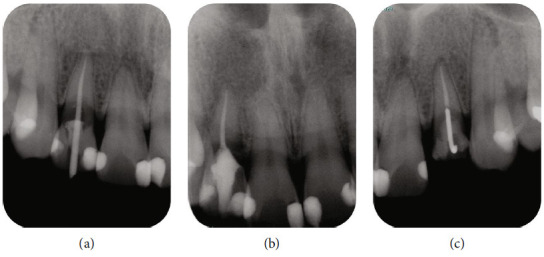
Radiographic images before intentional replantation. (a) Endodontic treatment was performed on the maxillary right lateral incisor after the pulp sensibility test result was negative. The working length was confirmed using a gutta-percha cone. (b) Intraoral radiographic image was taken immediately after the root canal filling with a single cone method using a methacrylate resin sealer (MetaSEAL soft paste). (c) An orthodontic hook was placed to assist intentional replantation with minimal damage to the periodontal ligament.
